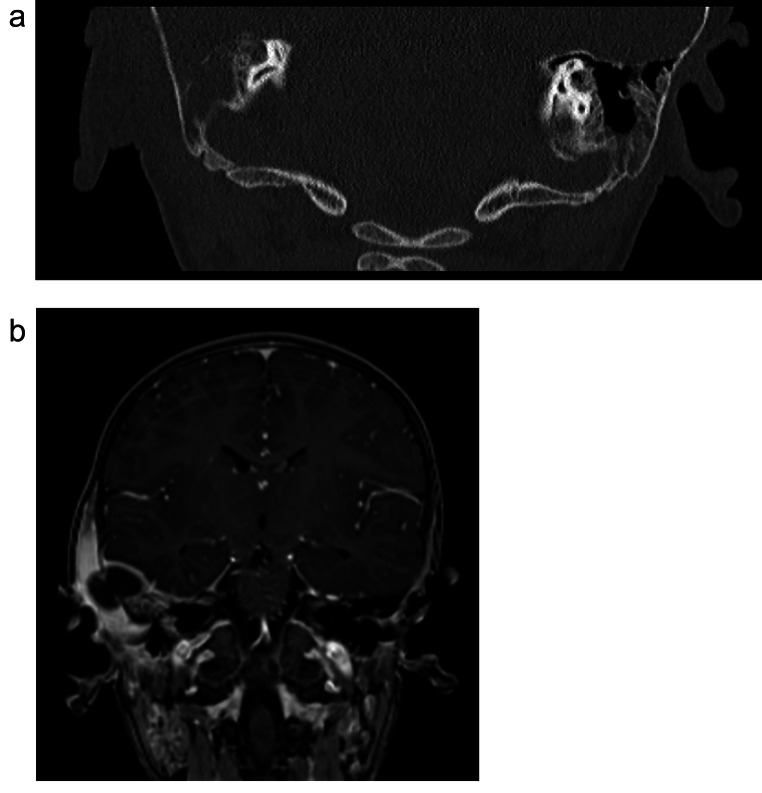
A: Coronal CT brain cut bone window showing complete opacification of mastoid air cells, erosion of mastoid air cell bony septum, mastoid cortex destruction and irregularity, and periosteal disruption. B: Coronal MR brain with contrast showing signs of pyogenic infection and coalescent mastoiditis, i.e. fluid collection, surrounding contrast enhancement, and periosteal disruption, subperiosteal/subcutaneous abscess
